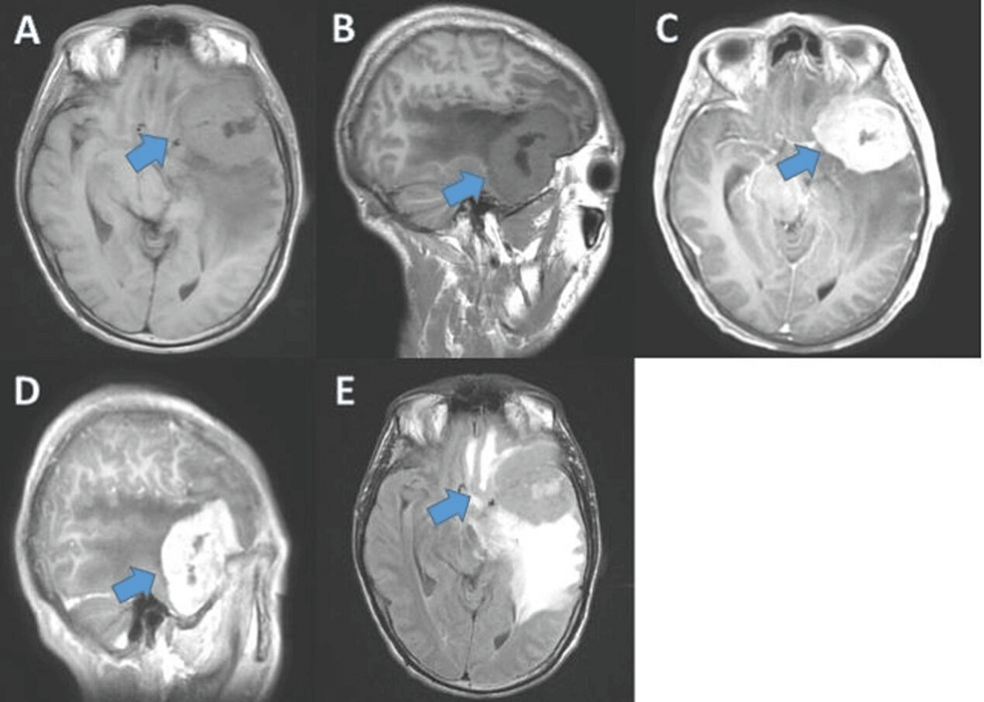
Brain MRI images: T1 sequence in axial section (A), sagittal section (B), injected axial section (C), and injected sagittal section (D); and T2 sequence in axial section (E), showing a left extra-axial frontotemporal process. MRI, magnetic resonance imaging
